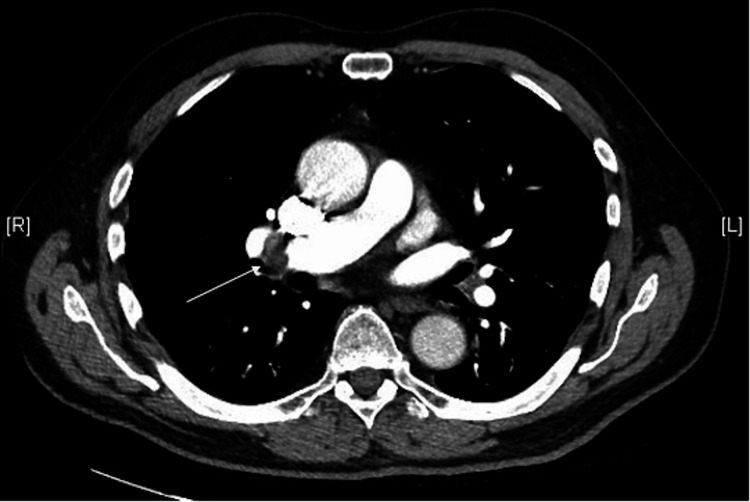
Image of the patient's pulmonary embolism as assessed by chest computed tomography
